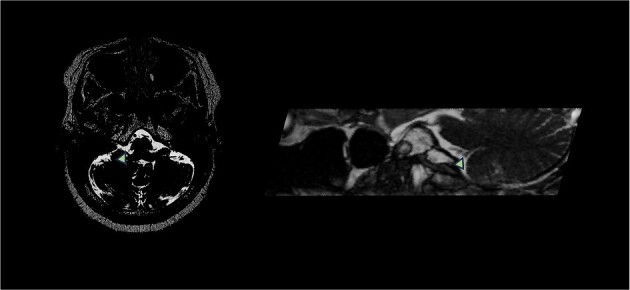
T2-weighted MRI with FIESTA sequence demonstrated right PICA compression of the right
